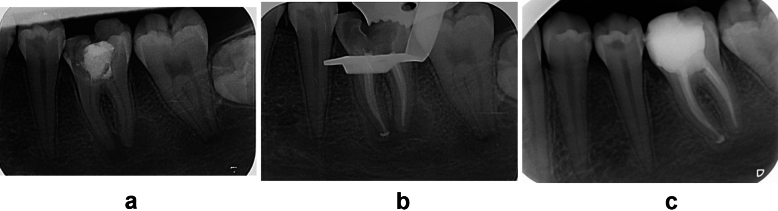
(a) Initial periapical radiograph showing periapical lesion. (b) Radiograph obtained following obturation and (c) Radiograph showing a healing periapical lesion at the 12-month recall.
